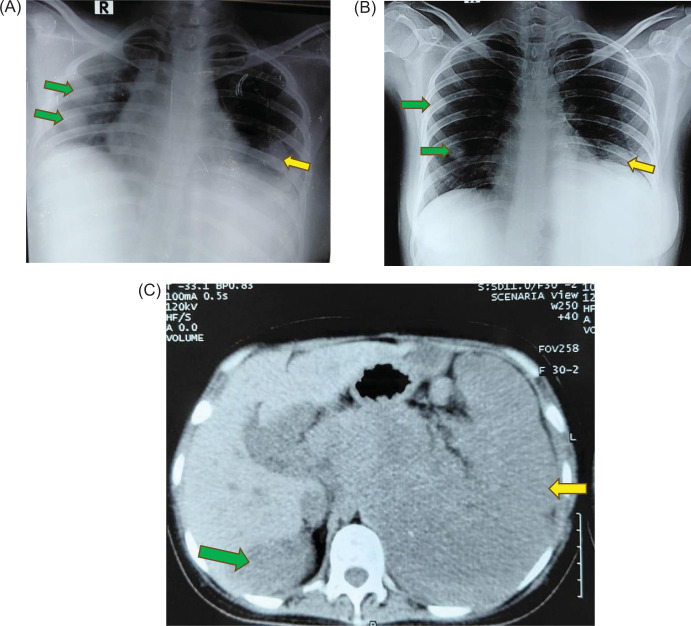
PET CT scan after 2nd line of chemotherapy in January 2026 showed the distribution of left abdominal mass (Yellow Arrow), liver metastasis (Green Arrow) and lung metastasis (Blue Arrow).
